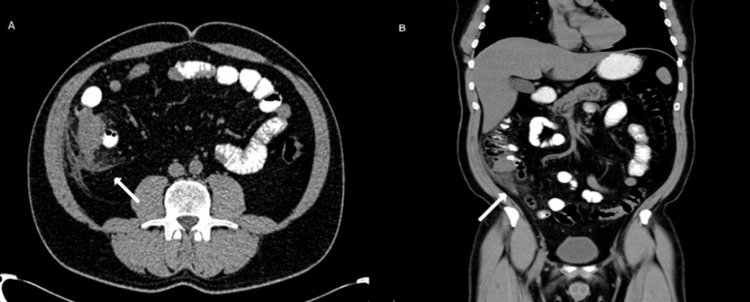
Abdominal CT scan Figure A: Axial view of abdominal CT scan: Inflammatory changes can be observed around the pericecal fat with thickening of the area. Figure B: Coronal view of abdominal CT scan: Loss of pericolic fat definition at the level of the cecum is evident (arrows).
